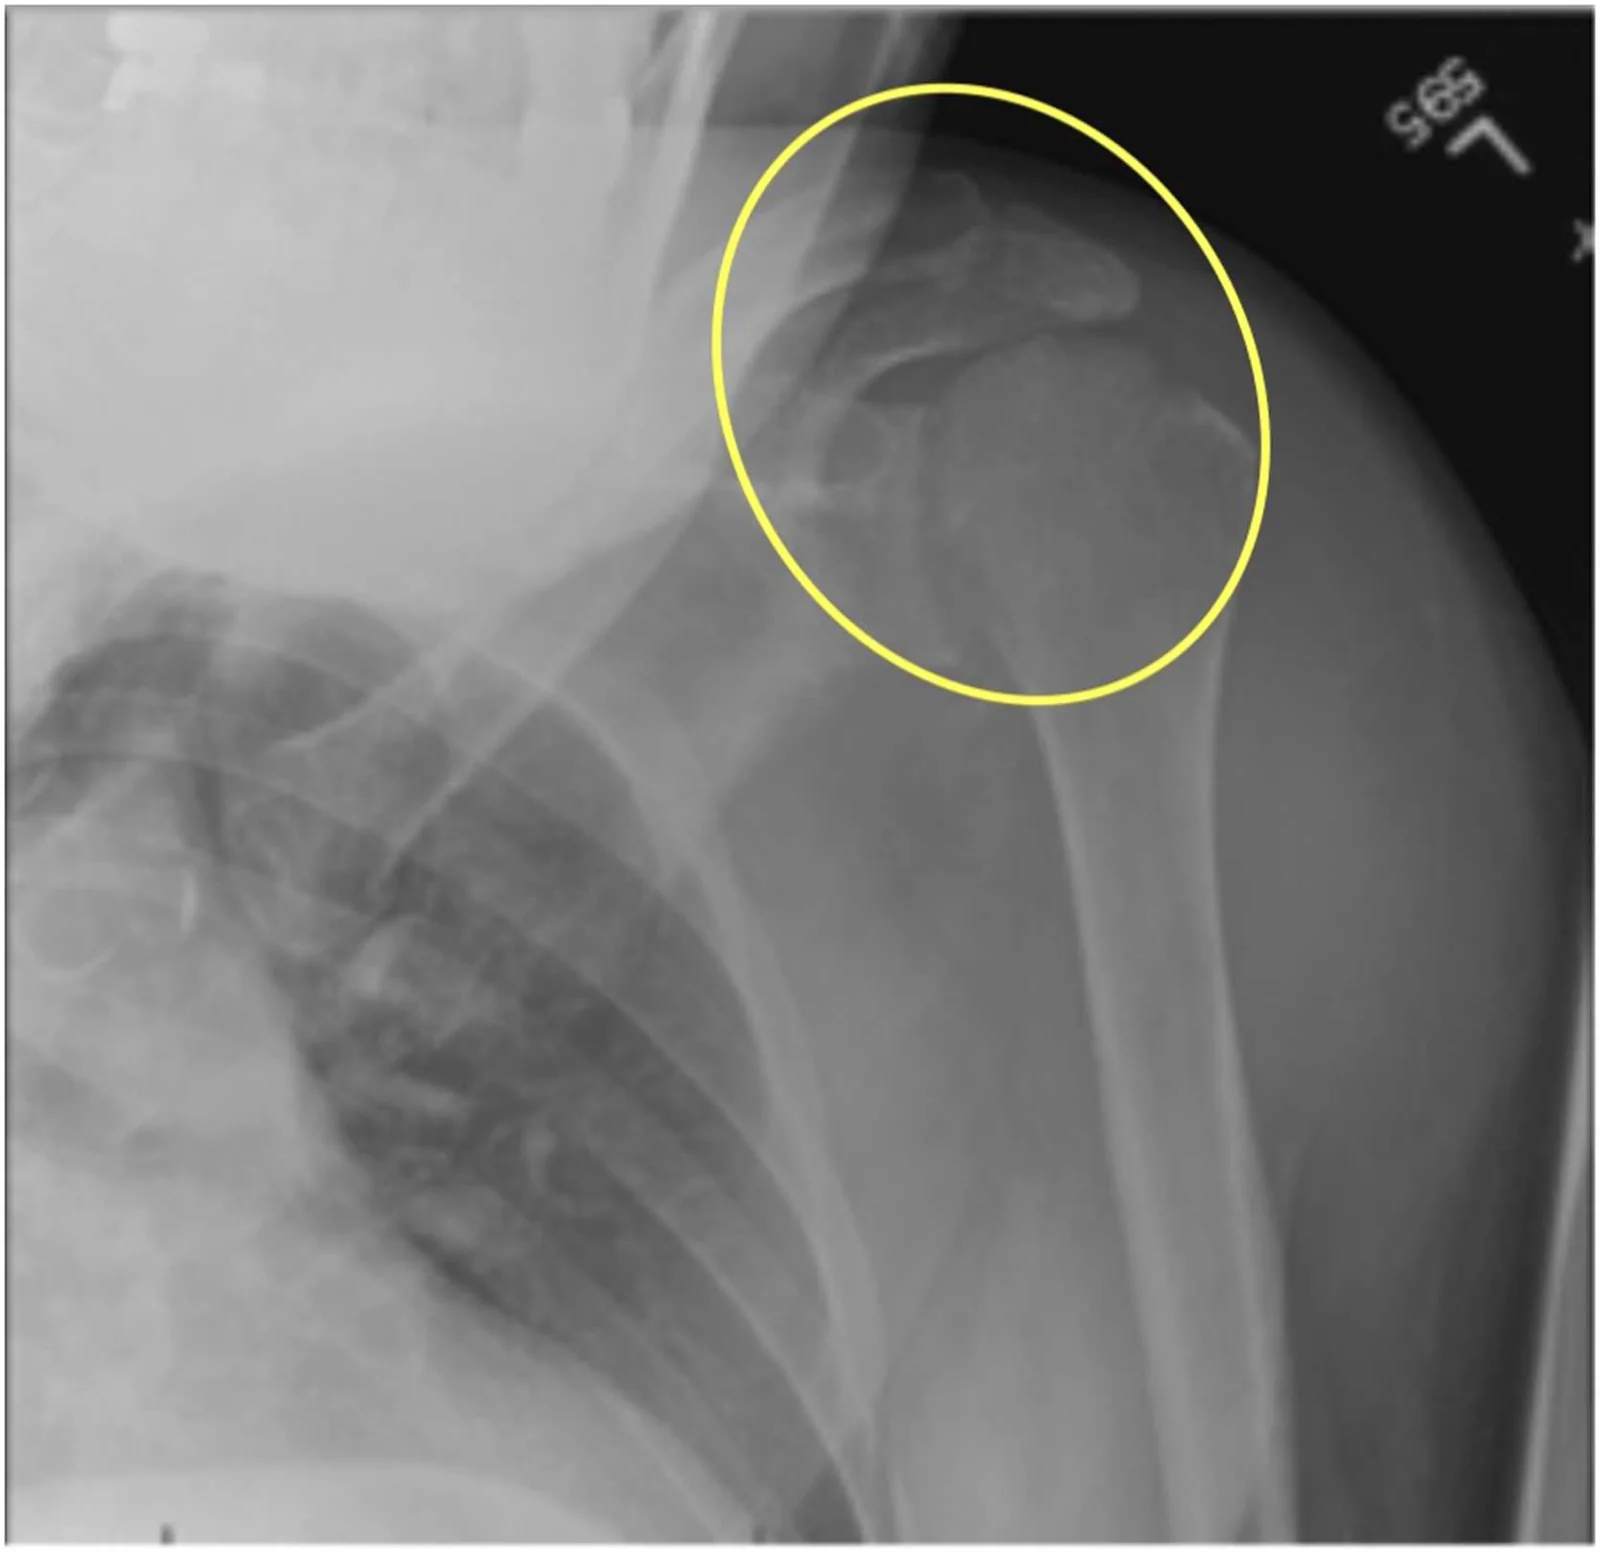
X-ray of left glenohumeral joint showing marked joint space narrowing with osteolysis of the humeral head and glenoid, and fragmentation at the inferior glenohumeral ligament.
